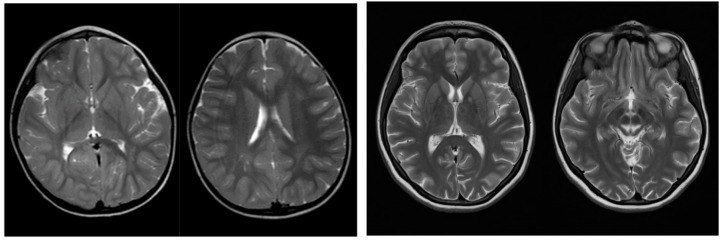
Axial T2-weighted magnetic resonance images of P1 (left panel) and P2 (right panel), respectively. For P1, zones of late myelination were observed (second image of the left panel), whereas for P2 T2 hyperintensities resembling Panda sign could be discerned (second image of right panel).
